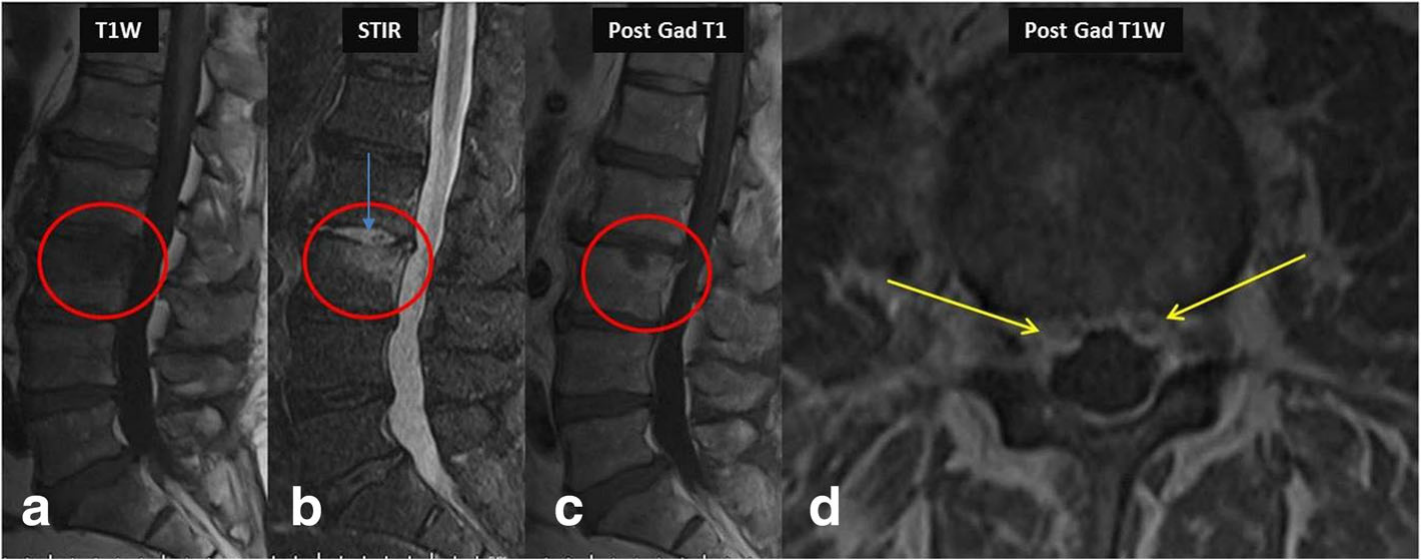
42-year-old-diabetic-male patient presented with low back pain for 2 weeks. MR imaging showed L2–3 vertebral endplate marrow edema (*red circle* in a, b and c) and increased fluid signal in the L2–3 intervertebral disc on STIR sequence (*blue arrow* in image b). Post contrast images (c & d) show enhancing soft tissue in the epidural space (*yellow arrows*), consistent with phlegmon, which is a very early complication. Post contrast imaging can help in differentiating between phlegmon, which does not contain non-enhancing or liquefied components, from an abscess which does have those components. *Streptococcus pneumoniae* was the causative organism
